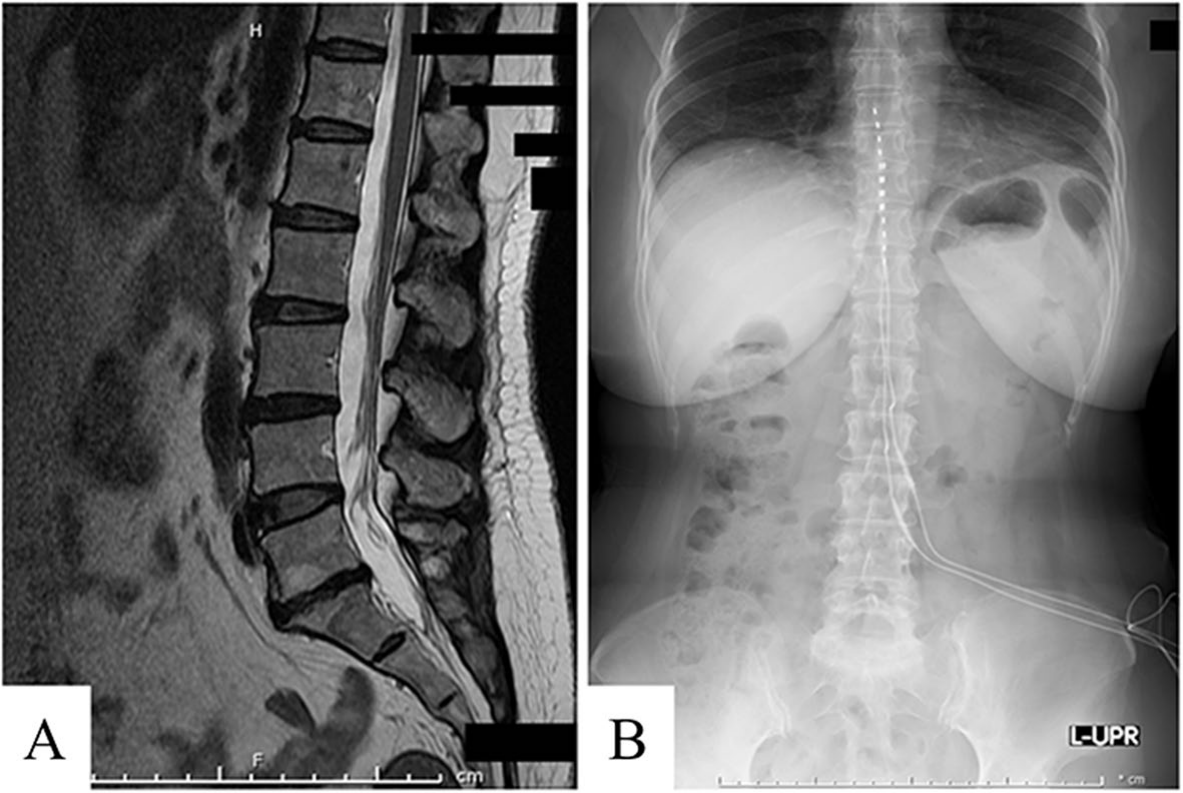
Preoperative and postoperative photographic images of Case 1. A Preoperative magnetic resonance image of the lower spine. Spinal canal stenosis was not observed. B Abdominal radiograph after SCS placement
